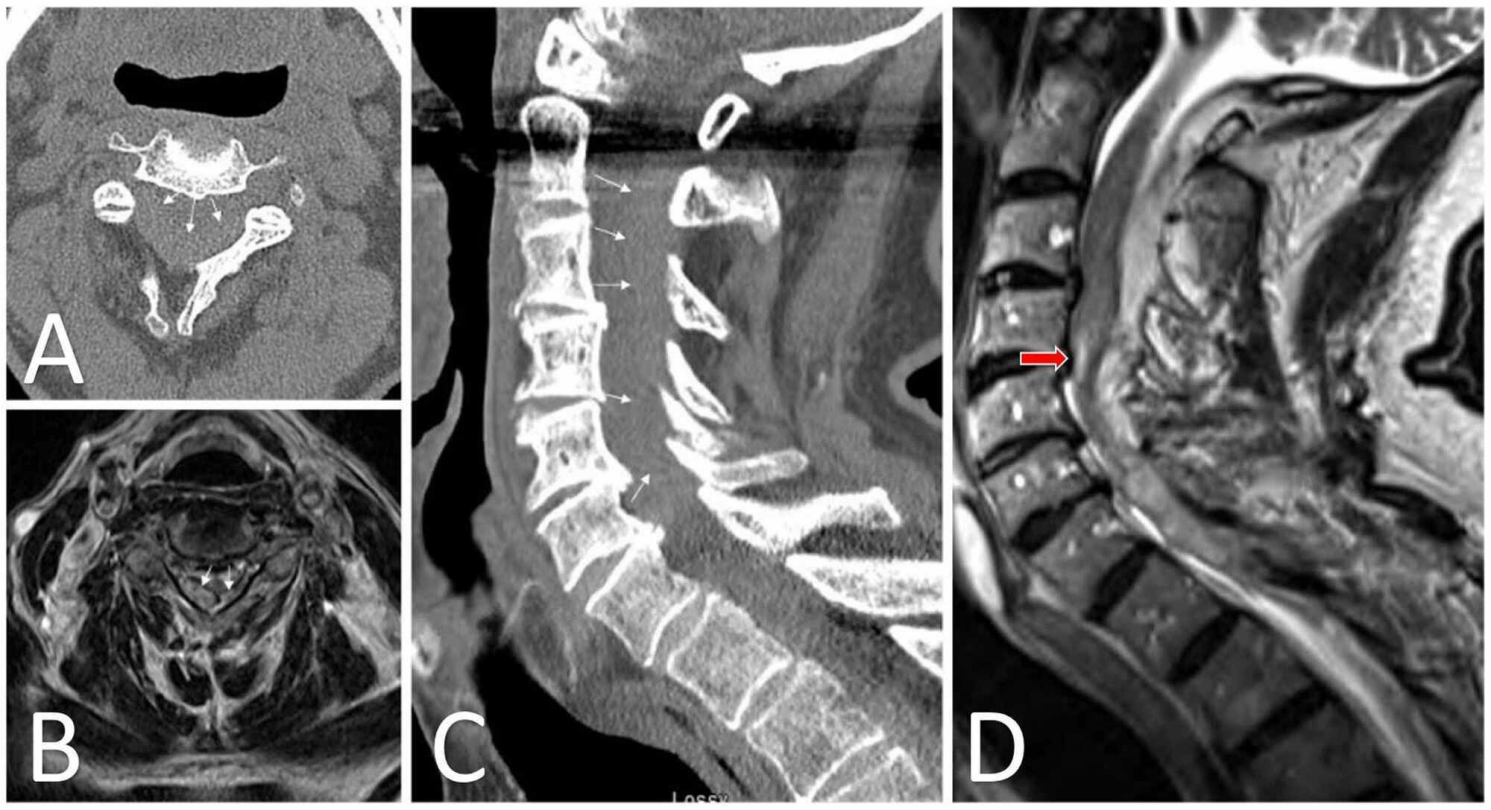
Acute epidural hematoma spanning from C2-T1 Axial (A) CT and (B) T2-weighted MRI demonstrating evidence of epidural fluid collection with central canal stenosis and spinal cord compression. Sagittal (C) CT and (D) T2-weighted MRI in the same patient. White arrows demonstrate the hemorrhage border with the spinal cord. Spinal cord signal change is evident adjacent to the C4-5 disc space (red arrow, D).
